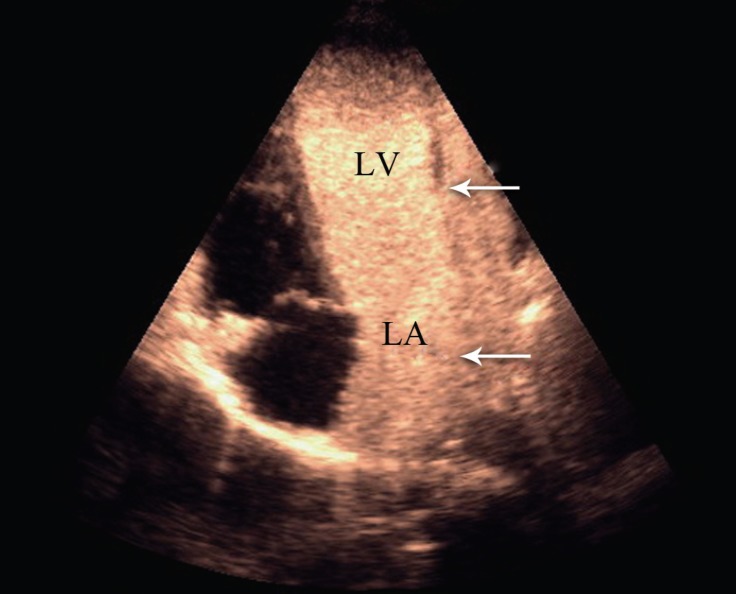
Transthoracic echocardiography in the apical 4-chamber view with contrast study, showing opacification of the left cardiac chambers (arrow heads) after agitated saline injection via the left brachial vein.
